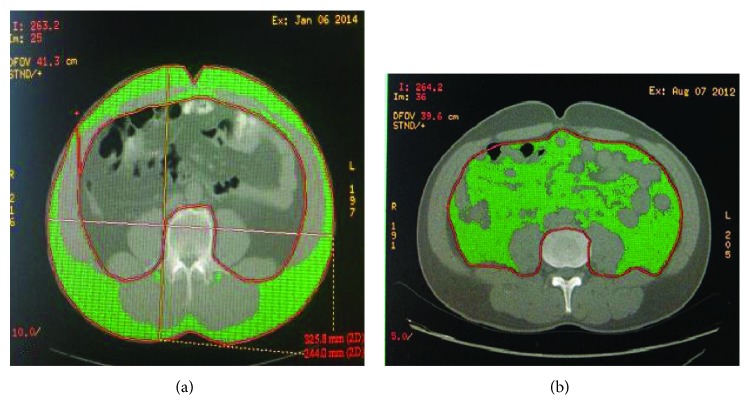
Images demonstrating the method used to determine abdominal fat, distribution on a CT scan obtained at the navel level: (a) the green area represents subcutaneous fat; (b) the green area represents visceral fat area.
